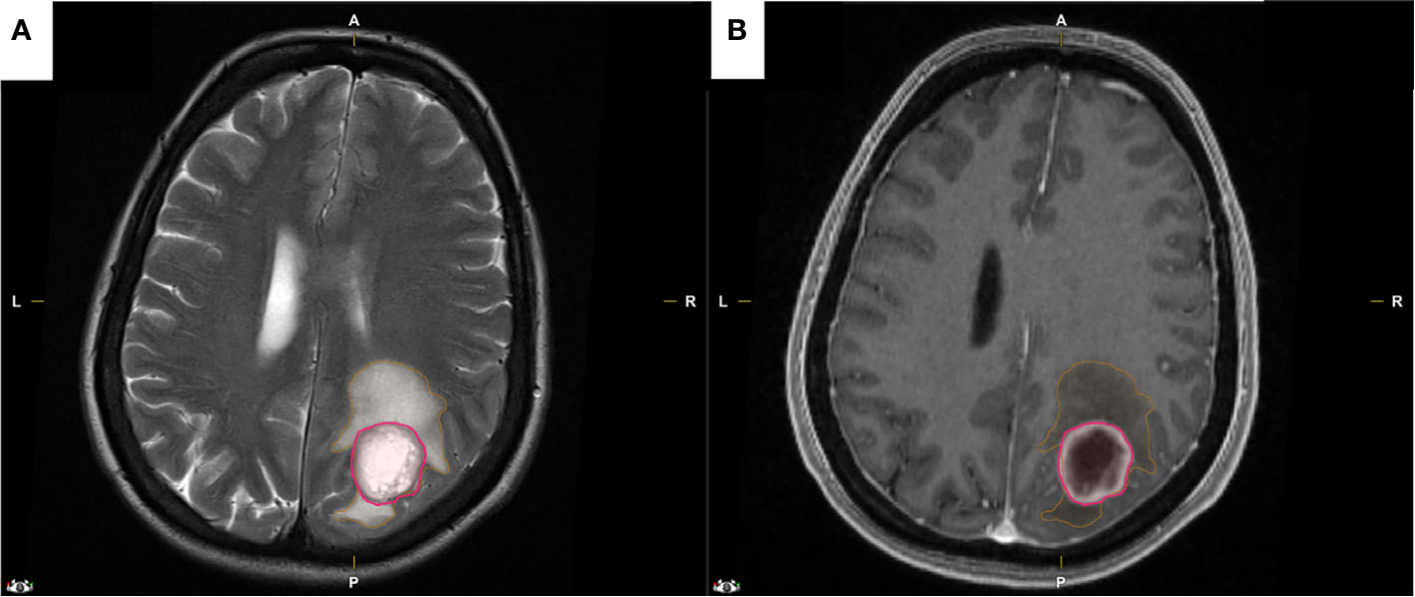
Representative MRI images that were used for the semi-automatic segmentation with IPlannet 3 software by Brainlab®. (A, B) Preoperative axial T2 and gadolinium enhanced T1 weighted MRI of a GBM patient with peritumoral edema encircled in orange and with tumor enhancement encircled in pink.
